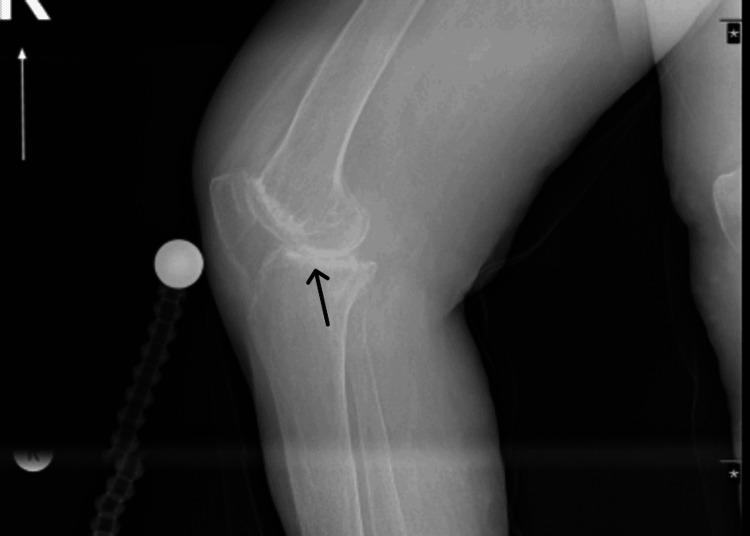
Plain radiography, lateral, weight bearing view, demonstrating bone on bone arthritis of the medial compartment.
